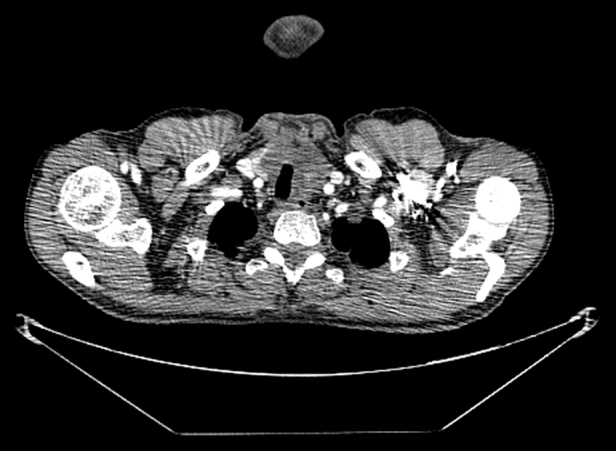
CT scan of the first patient, showing a nodular mass with dystrophic calcification.
